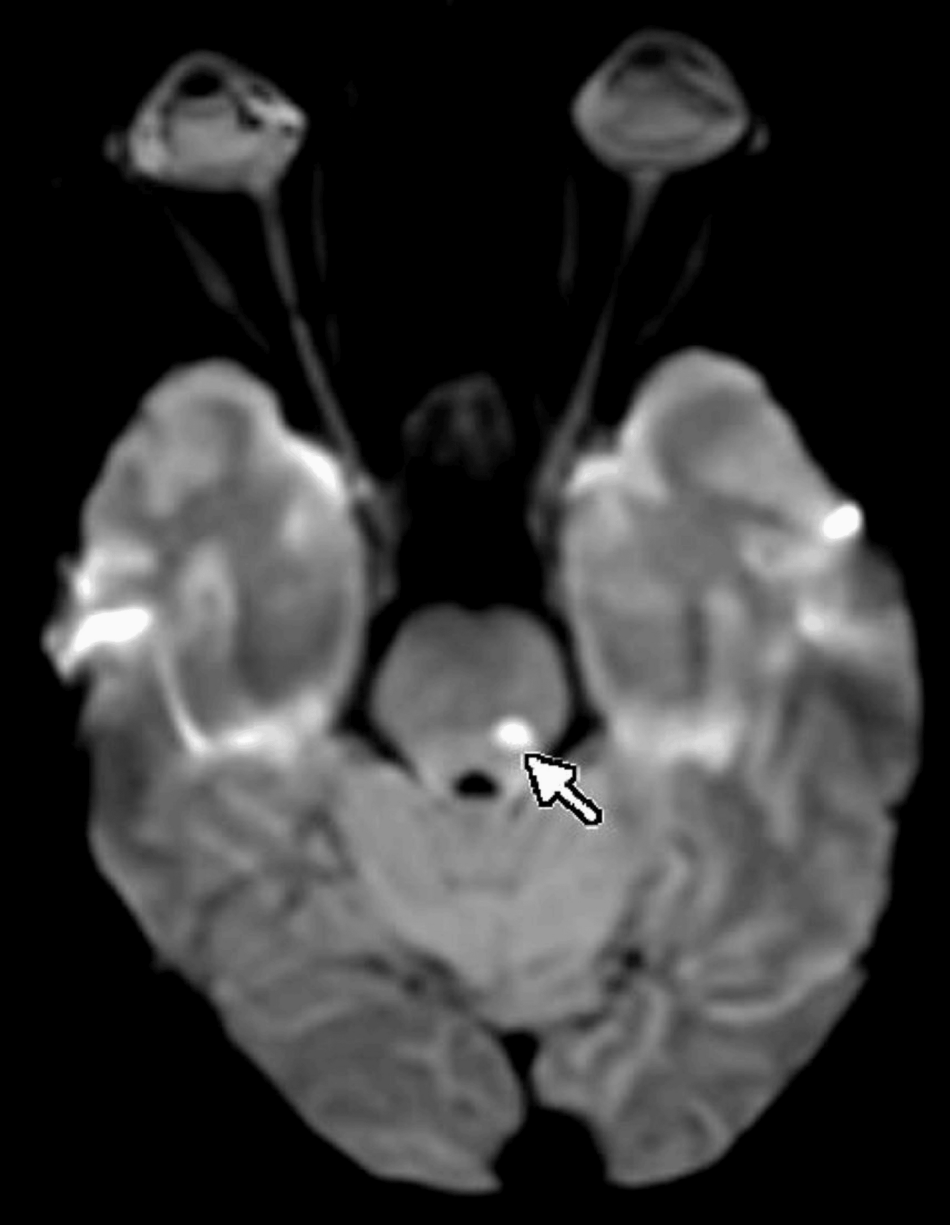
MRI of the brain revealed a high-signal nodule, approximately 5 mm in diameter, located in the left pons on DWI (marked with an arrow). DWI: diffusion-weighted imaging
